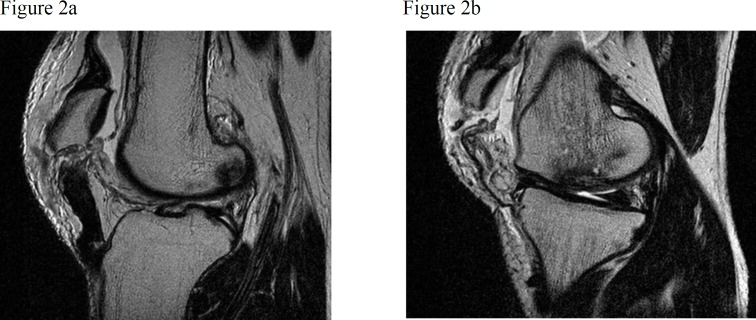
(a) MRI of bilateral rupture of patellar tendons in left knee (b) MRI of bilateral rupture of patellar tendons in right knee.
